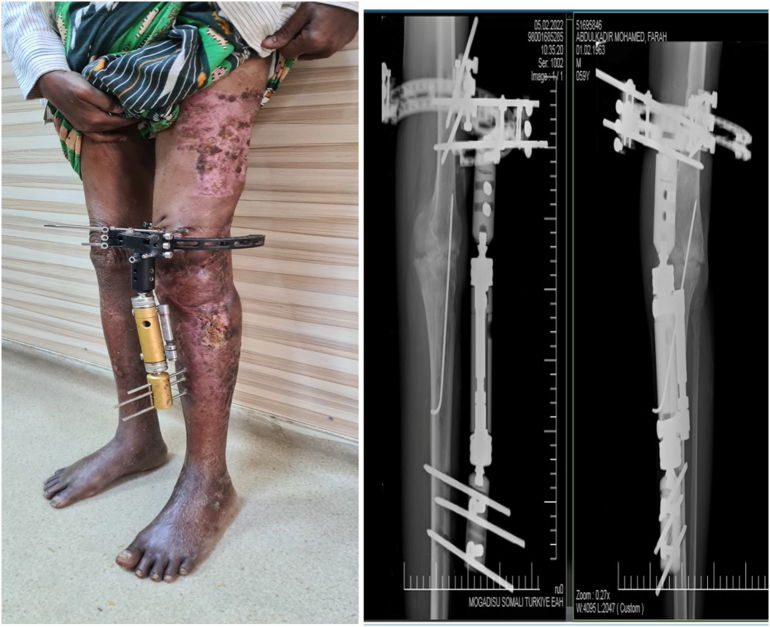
Three months clinical and radiographs follow-up of the knee showing good alignment and excellent healing of the flap without any signs of infection.
